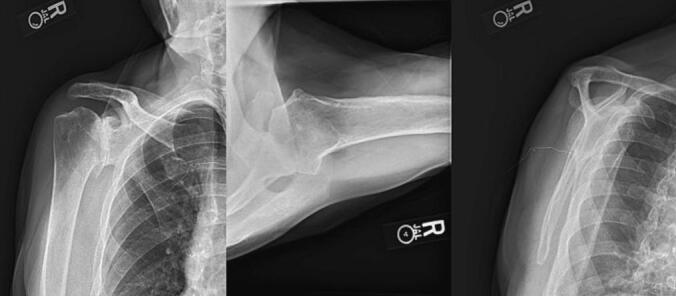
Pre-operative AP, axillary, and scapular radiographs of right shoulder demonstrating severe glenohumeral osteoarthritis in patient I.
